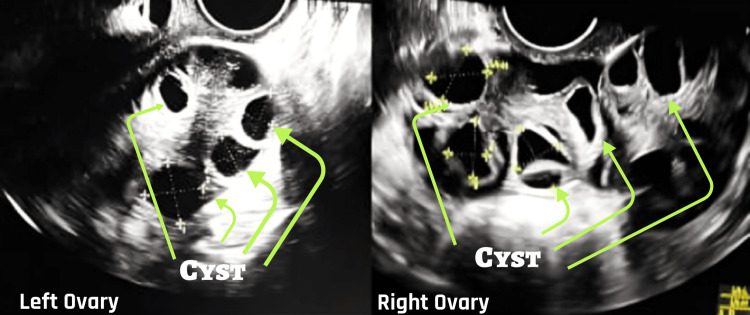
Transvaginal sonography image of patient's right and left ovary on day 2 of menstrual cycle Depict multiple cysts present in the right and left ovary.
